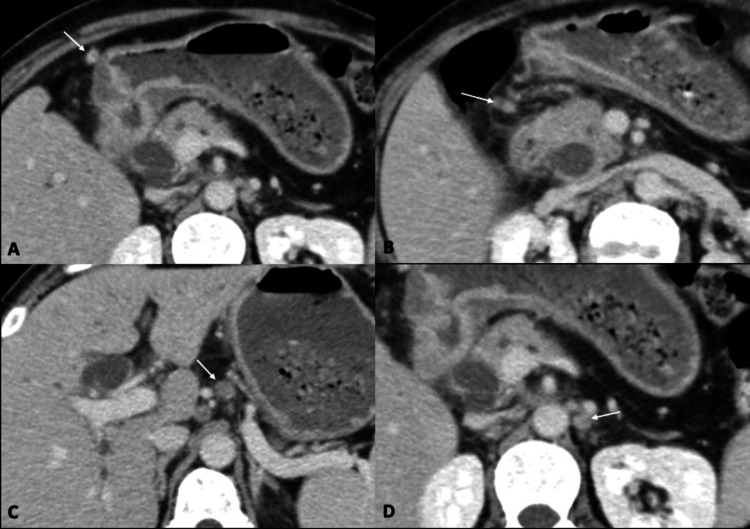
Lymph nodal spread Axial sections of the CECT of the abdomen show enhancing lymph nodes in the greater curvature (A), infrapyloric (B), celiac (C), and para-aortic (D) regions. Interestingly, except for the greater curvature, all other stations show clusters of lymph nodes. CECT: contrast-enhanced computed tomography
